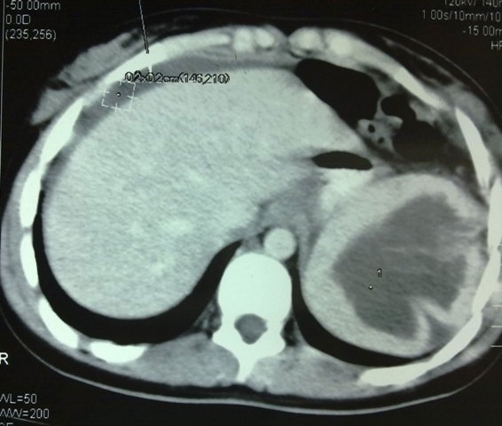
CT scan showing the ruptured cyst.
